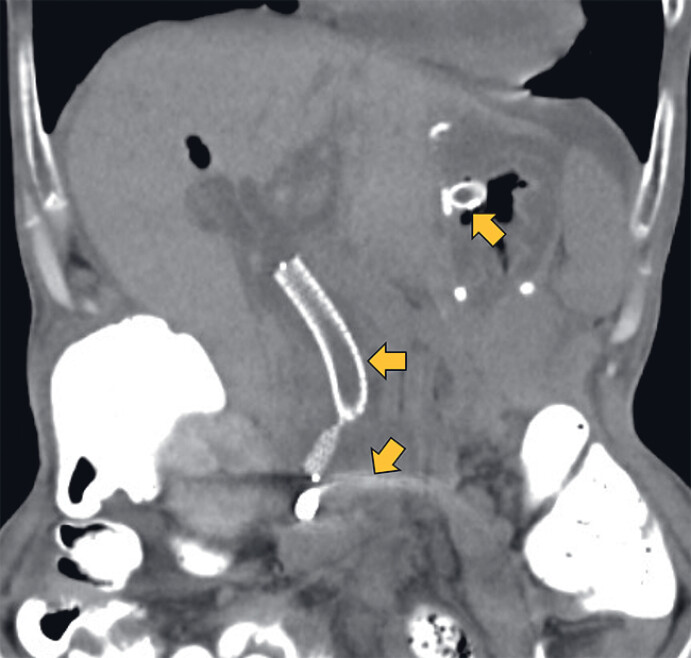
Follow-up computed tomography demonstrates the disappearance of the afferent loop and bile duct dilatation. Orange arrows indicate the stents.
